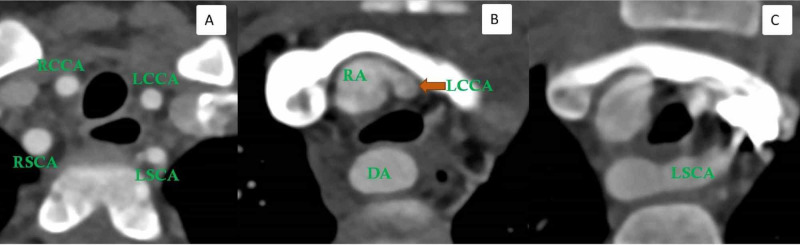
Double aortic arch with atresia between the left common carotid and the left subclavian artery in a two-year-old male child Axial CTA image (A) shows four aortic branches in a relatively symmetrical presentation seen just above the aortic arch (four-vessel sign). Axial CTA image (B) shows the posterior course and tenting of the left common carotid artery (arrow). Axial CTA image (C) shows the left subclavian artery coursing behind the esophagus and coursing from right to left. RSCA: Right subclavian artery; LSCA: Left subclavian artery; RCCA: Right common carotid artery; LCCA: Left common carotid artery; DA: Descending aorta; RA: Right arch; CTA: Computed tomography angiography; MRA: Magnetic resonance angiography
